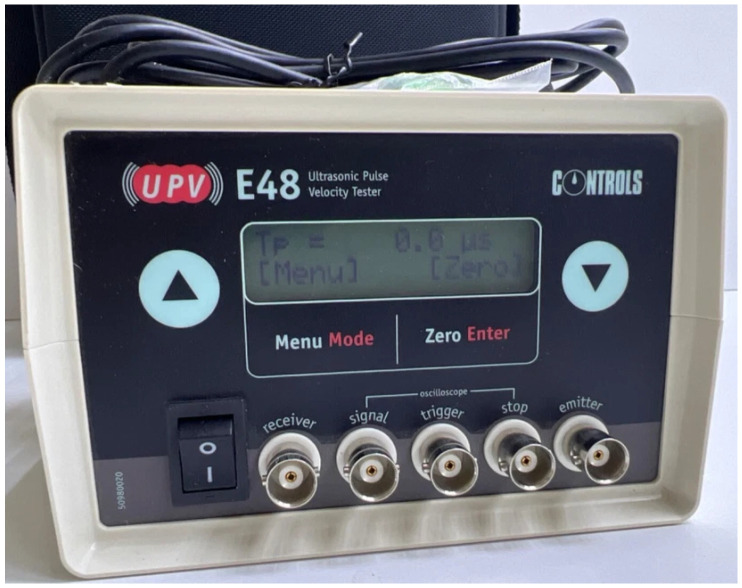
Ultrasonic pulse velocity (UPV) measuring device [32].
